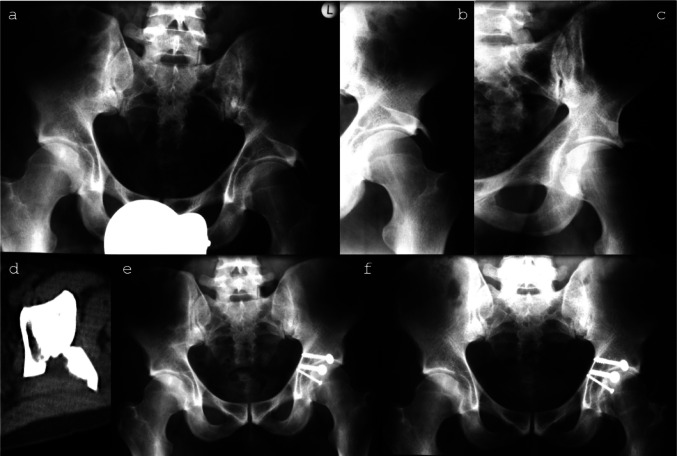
33 year-old male after MVA sustaining a moderate traumatic brain injury and a dashboard injury with a large posterior wall fracture without hip dislocation. The conventional x-rays (a, b, c) show the isolated wall fragment at its typical location posterior and superior to the acetabulum. CT confirmed. the single large fragment (d). After 10 days, ORIF was performed using the Kocher-Langenbeck approach and three lag screws were used (e). 3-year follow-up showed radiological uneventful healing. The clinical result was graded very good (Merle d´Aubigné Score 17 pt, slight groin pain, normal hip range of motion, no nerve deficit)
